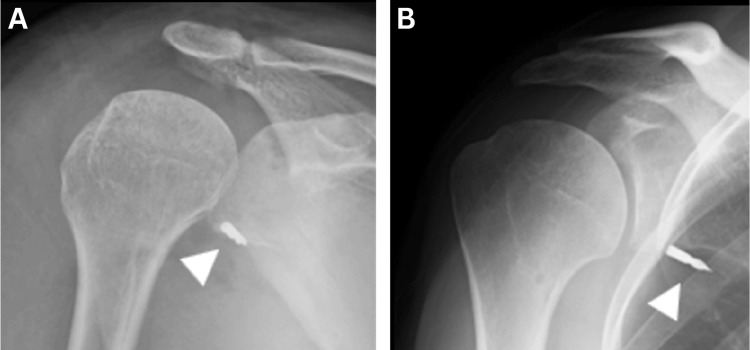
X-ray images taken soon after operation (A) and three weeks after surgery (B) The drill tip seemed to remain in the glenoid soon after surgery. However, three weeks later, the drill tip was moved.
